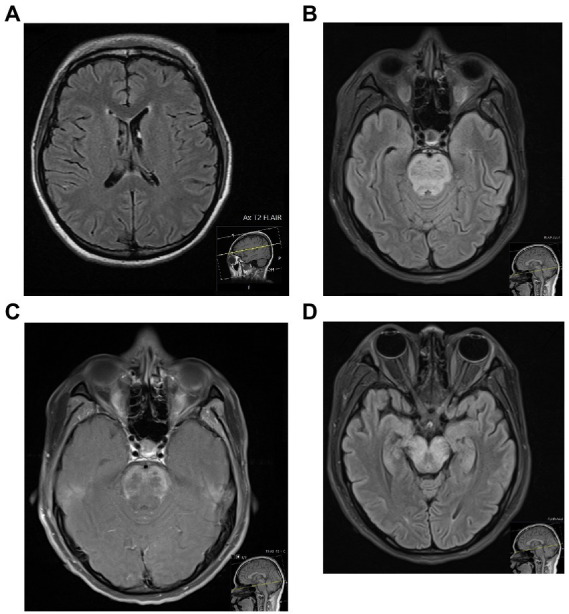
Two patients with NPSLE, who both presented with seizures in the context of a systemic hyperinflammatory syndrome. The transaxial MRI FLAIR of the first patient (A), a 27-year old female, was unremarkable. The MRI of the second patient (B–D), a 20-year old female, showed diffuse swelling of the pons with increased T2/FLAIR signal (B) and diffuse peripheral abnormal contrast enhancement (C), with extension of the T2/FLAIR signal inferiorly to the lower medulla and superiorly across white matter tracts of the midbrain (D) – all of which were in keeping with a severe CNS vasculitis.
